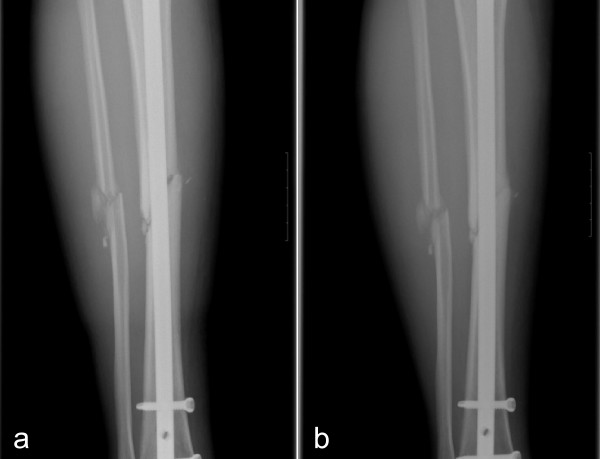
The right tibia AP radiographs of a 47-year-old male patient before osteoblast injection (a) and eight weeks after injection (b) into the fracture of the tibia shaft.
